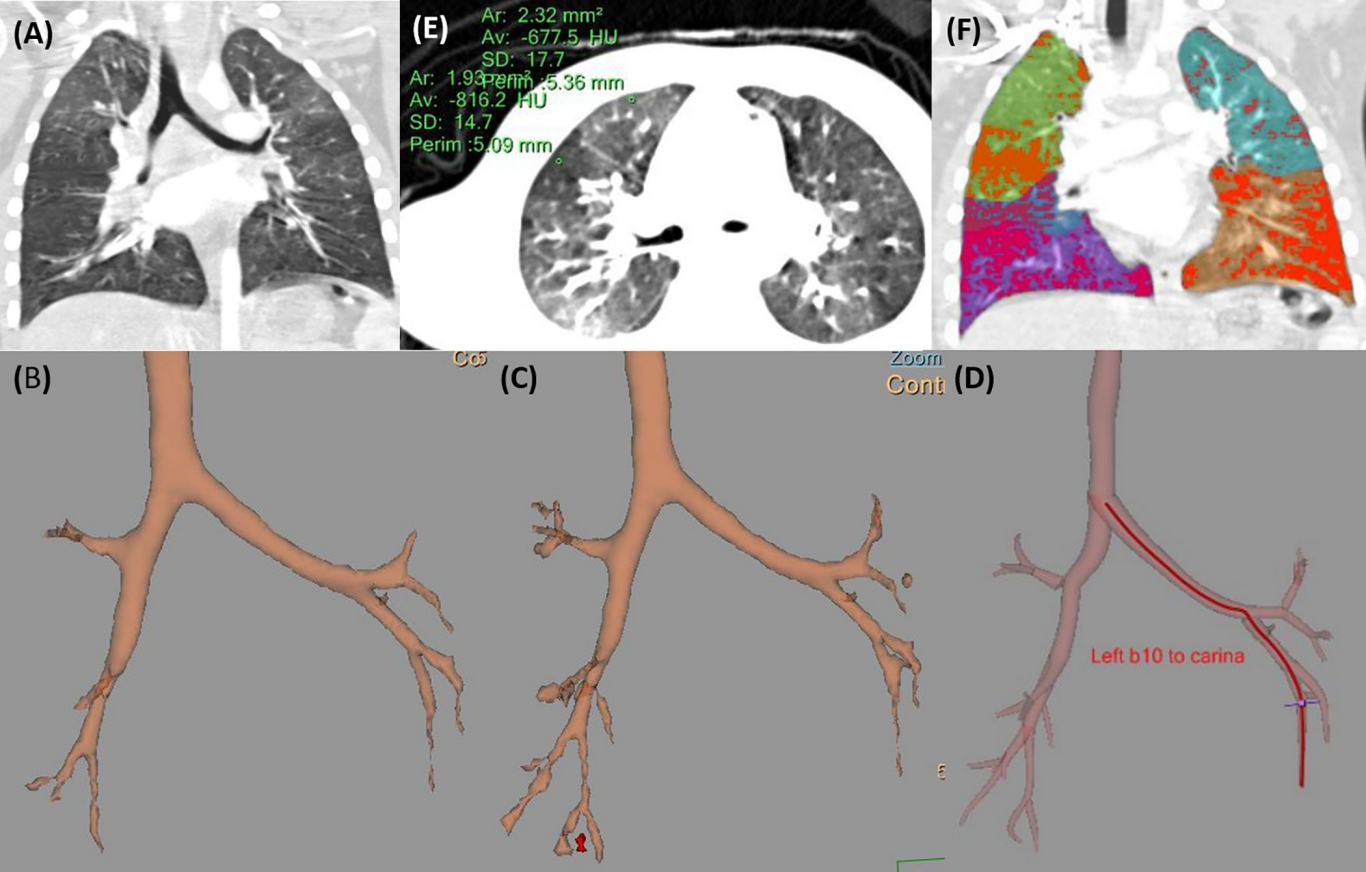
A representative case of successful quantitative analysis of a four-year-old girl with post-infectious bronchiolitis obliterans. (A) The coronal reconstructed CT image demonstrates bronchial wall thickening with mosaic attenuation in bilateral lungs. (B and C) Automatic airway segmentation (B) and subsequent manual editing (C) reveal all segmental bronchi in bilateral lungs. (D) Each segment is labeled. (E) Small regions of interest are drawn at normal and obviously air-trapping lung parenchyma to calculate the individualized threshold value of air-trapping. (F) Lung parenchyma area with CT attenuation value lower than the calculated threshold is marked with orange.
